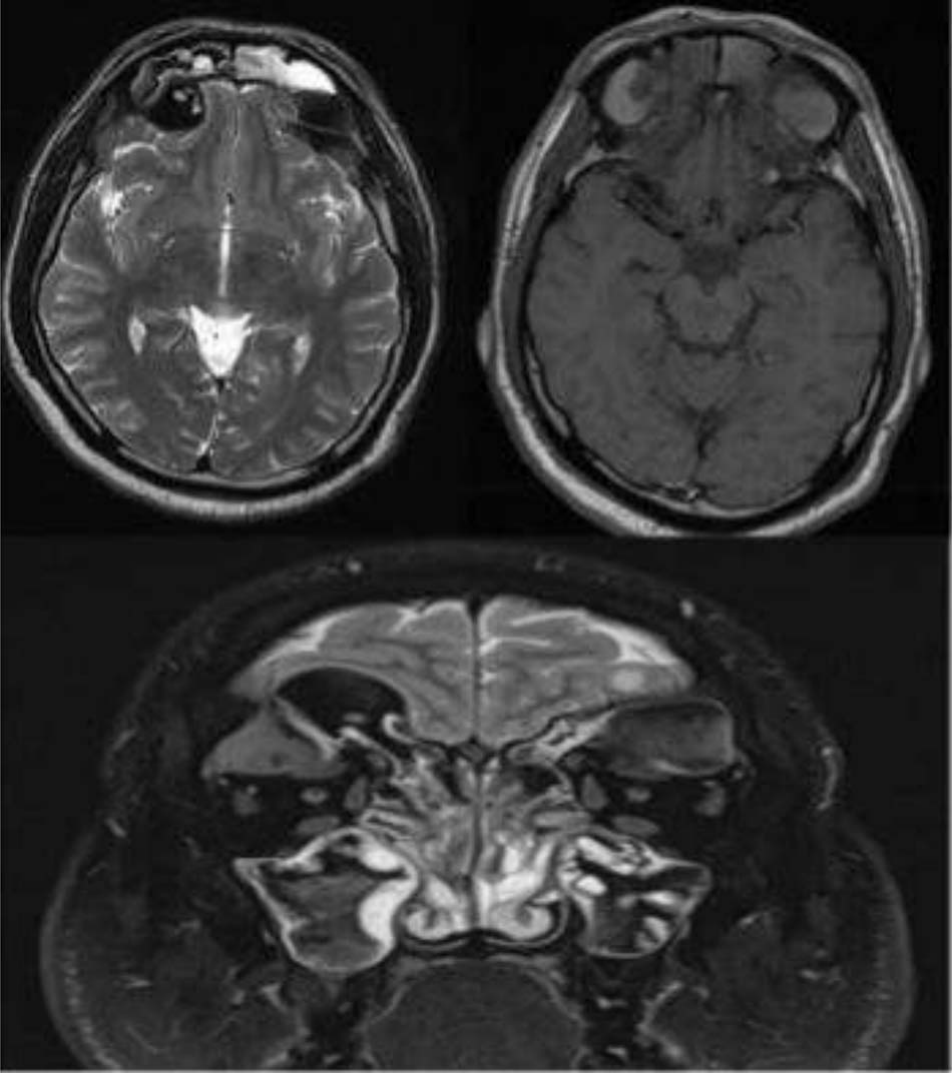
MRI paranasal sinuses axial and coronal T2 and axial T1 The MRI shows the expansion with the remodeling of the sinuses and intra-orbital/intracranial extension. The hyperdense contents show marked low signal intensity on T2 and high T1 signal. No tissue invasion of the orbital or brain parenchyma.
